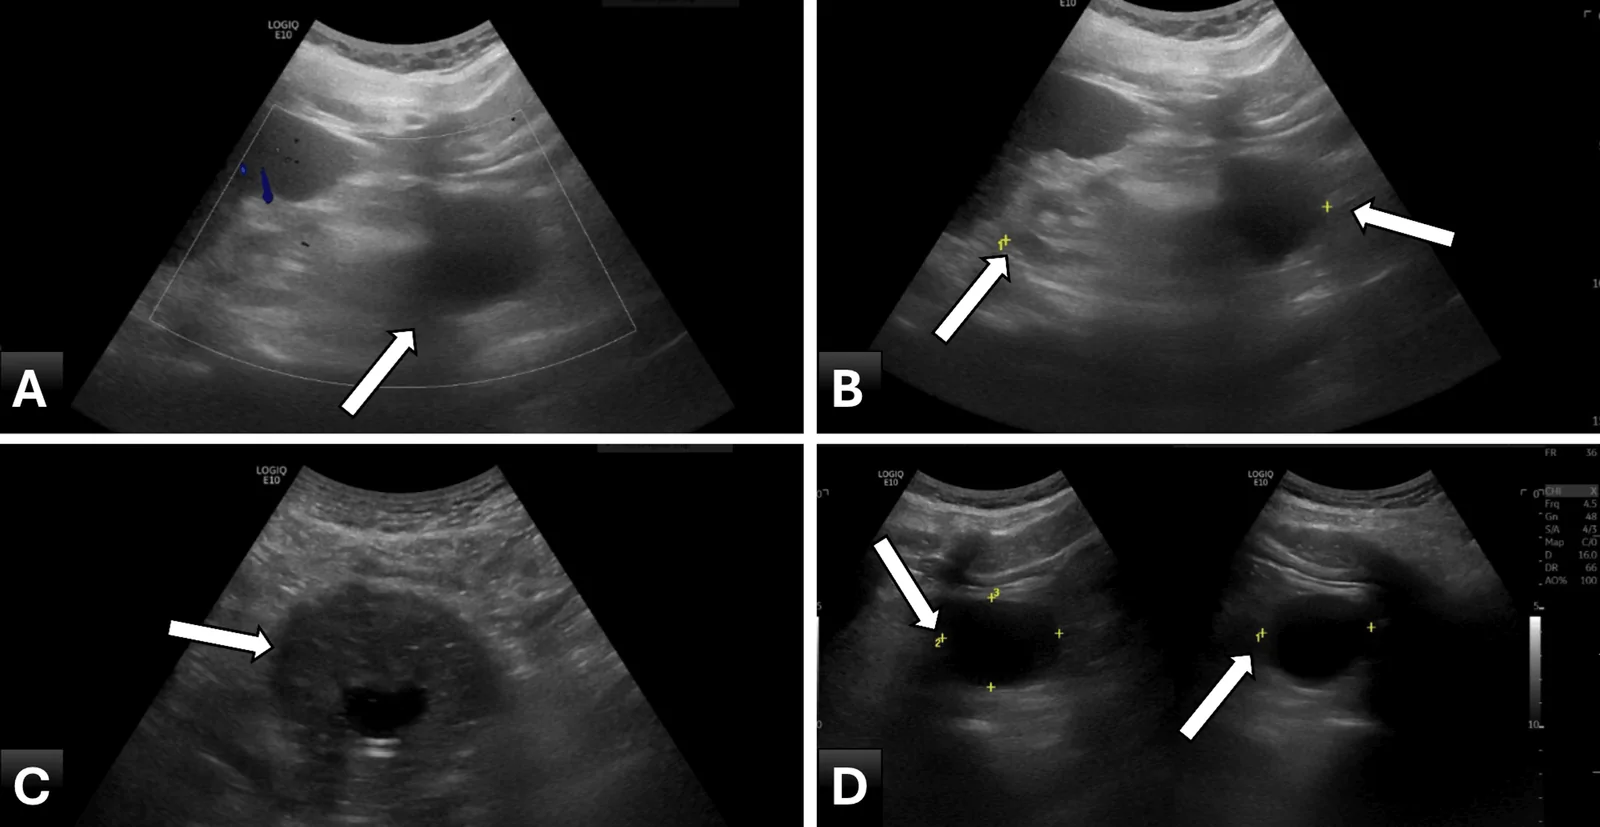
Renal ultrasonography demonstrating chronic obstructive uropathy of the left kidney (A) Longitudinal view demonstrating a simple left lower pole renal cyst (arrow). (B) Left kidney demonstrating severe grade IV hydronephrosis with marked cortical thinning (arrows). (C) Transverse bladder view showing diffuse concentric bladder wall thickening (arrow), consistent with chronic bladder outlet obstruction and/or chronic cystitis. (D) Additional orthogonal view of the simple left lower pole renal cyst (arrow).
